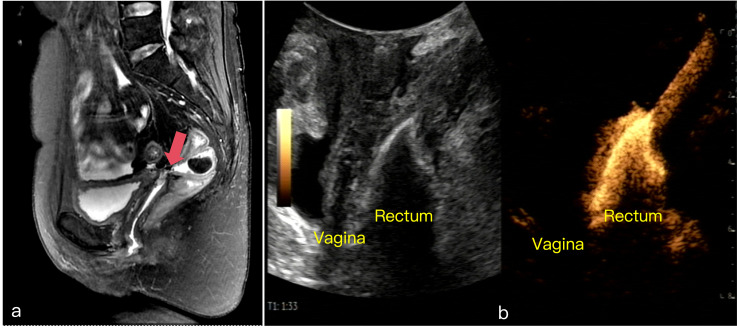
A case of rectovaginal fistula at the vaginal stump: MRI detection and UCAE miss. (a) Contrast-enhanced MRI in the sagittal plane clearly delineates a fistulous tract at the vaginal stump (arrow). (b) UCAE image showing no fistulous tract, likely due to its high anatomical location.
